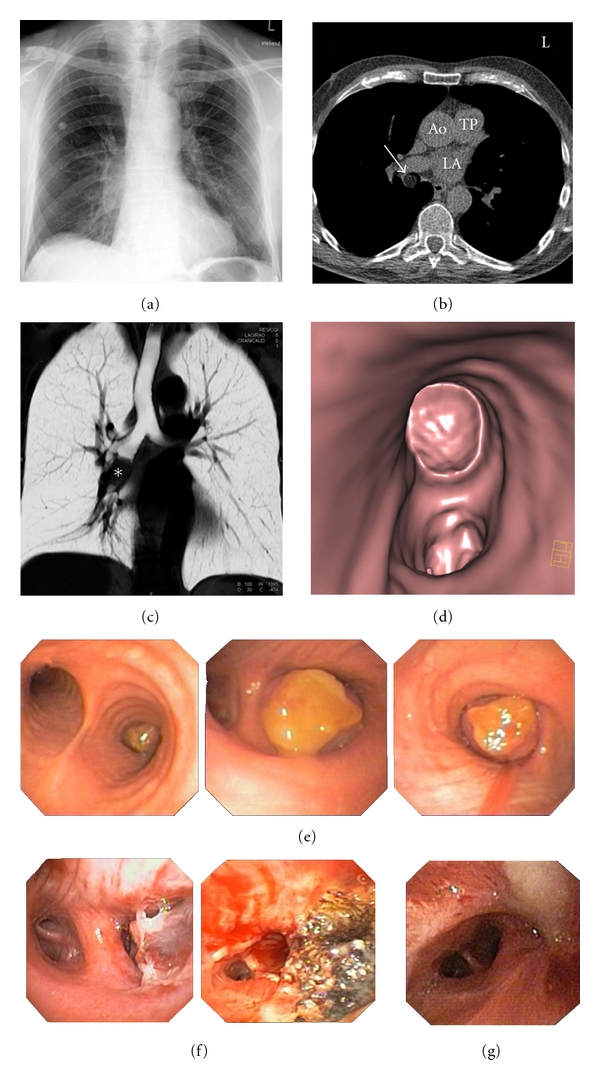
(a) On p.a. chest X-ray a prominent right hilum and a subtle increase in parenchymal density in the right infrahilar region was noted, a mass could not be clearly identified, however. (b) A noncontrast-enhanced CT scan of the chest revealed a mass within the bronchus intermedius (arrow) consisting mostly of fat with minimal inclusions of soft tissue density (Ao: Aorta ascendens; PT: pulmonary trunk; LA: left atrium). (c) Coronal slide in inverted thin-volume-rendering-technique of a contrast-enhanced CT scan performed for further workup demonstrated position and extent of the mass within the bronchus intermedius. (d) Virtual-bronchoscopy reconstruction from the nonenhanced CT scan displayed the mass within the proximal bronchus intermedius. The adjacent upper lobe bronchus was not affected. (e) Endobronchial round tumorous obstruction of the intermediate bronchus with a smooth surface with subtotal obstruction of the intermediate bronchus. (f) Recurent subtotal exclusion of the intermediate bronchus in the course of the disease and repetition of Argon laser therapy. (g) Incipient focal metaplasia of respiratory mucosa into squamous epithelium.
